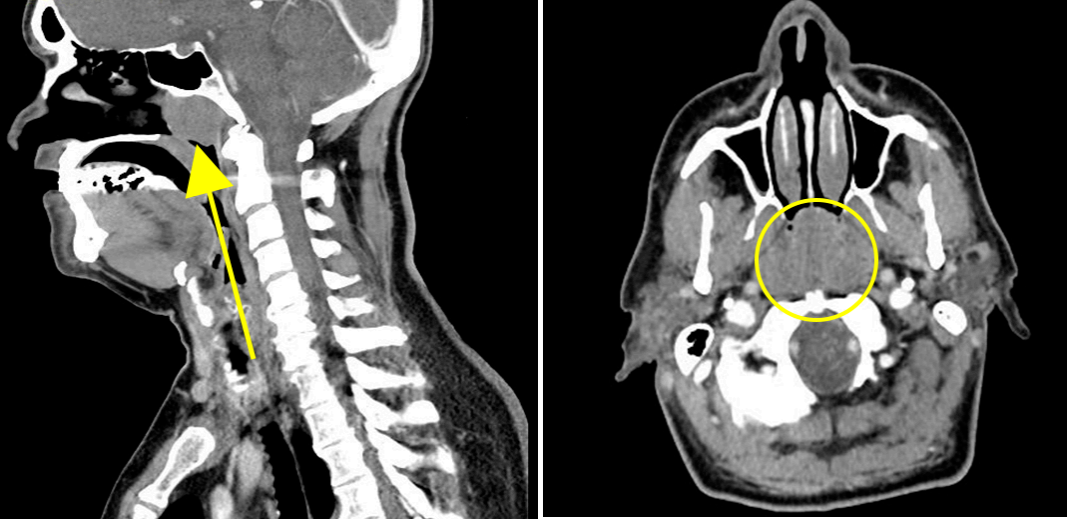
Left: Sagittal CT soft tissue window displaying 3.2cm x 3.0cm nasopharyngeal mass with complete obstruction of nasal airway. Right film shows axial CT with nasopharyngeal mass adjacent to nasal septum.
